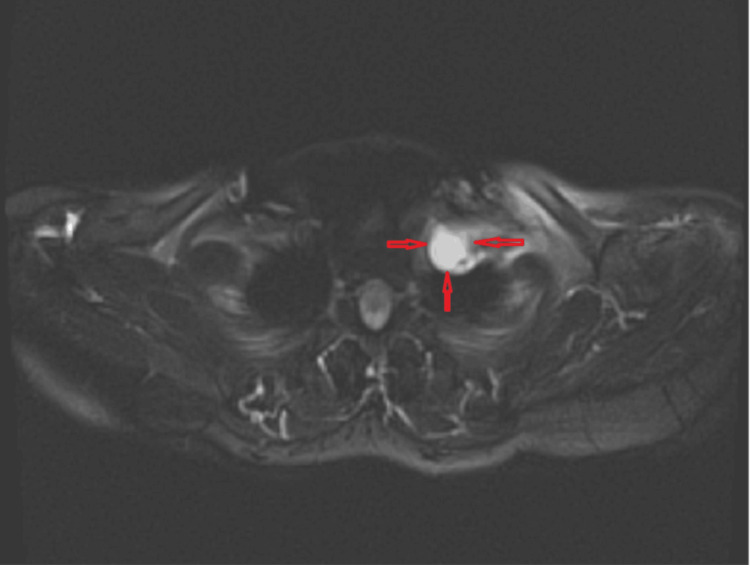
Axial section of magnetic resonance imaging showing the location and appearance of the lesion.
